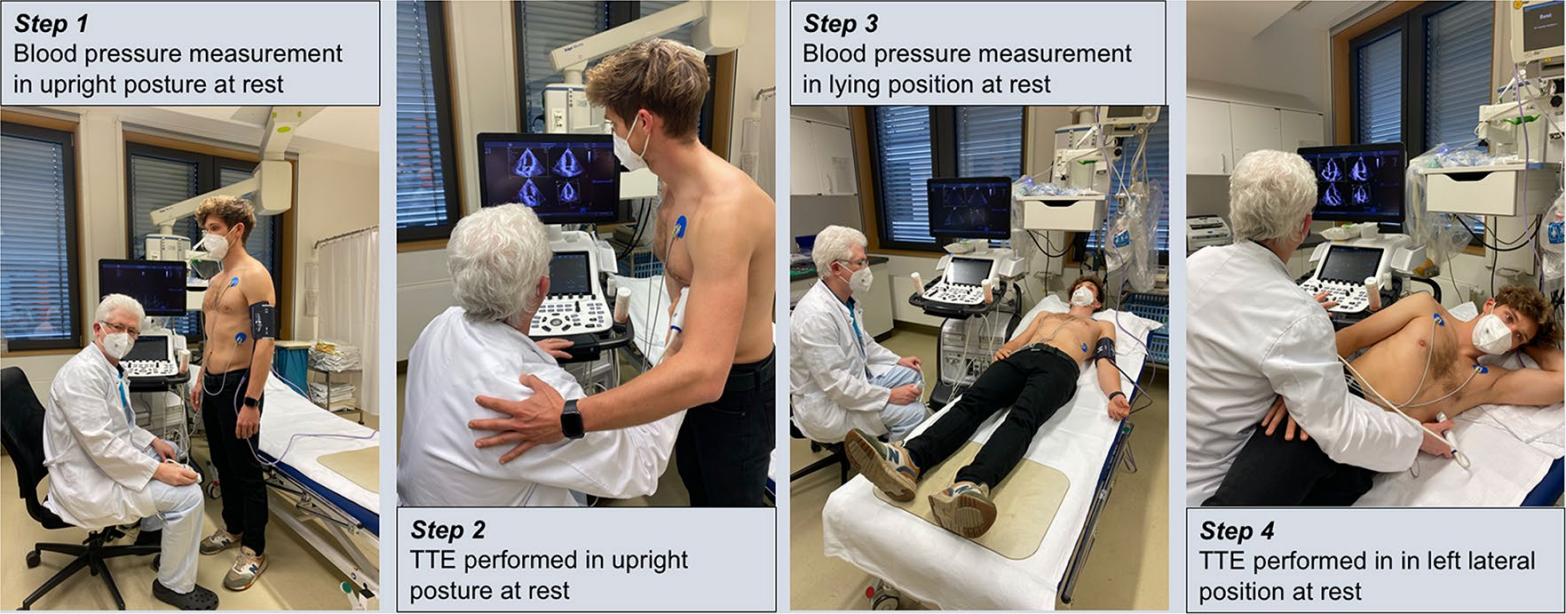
The diagnostic algorithm. *TTE *Transthoracic echocardiography
